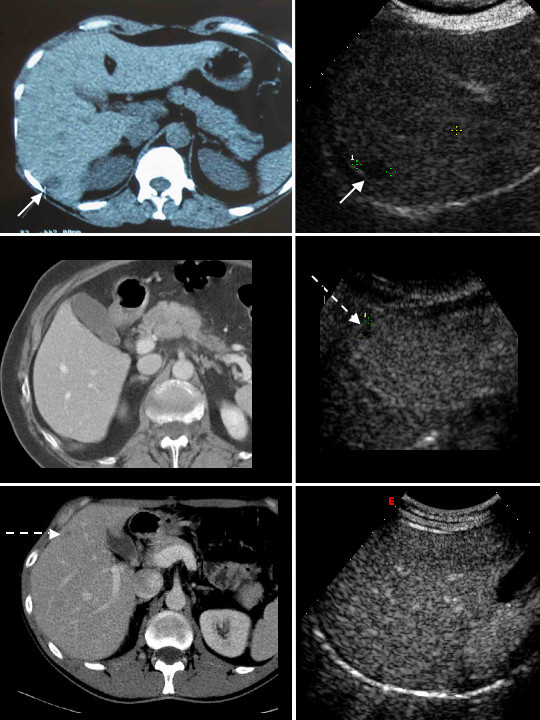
Comparison of CT (left column) and contrast enhanced US (CEUS) (right column) appearance of liver metastases in the venous-late phases of portal perfusion. In the upper row a concordance is shown in the detections of a subcapsular metatasis localized in liver segment 7. Metastasis appears hypodense at CT and hypoechoic at CEUS (continuous-line arrows). In the middle and lower rows, two cases of discordance between CT and CEUS are shown. In the middle row the tiny subcapsular metastasis (dashed-line arrow) was detected by US, but not by CT. The metastastic nature of the lesion was confirmed intraoperatively at the time of resection of the primary cancer plus metastasectomy. In the lower row, the metastatic lesion (dashed-line arrow), located anteriorly in segment 6, was identified by CT and missed by CEUS. The malignant nature of the lesion was confirmed at surgery.
